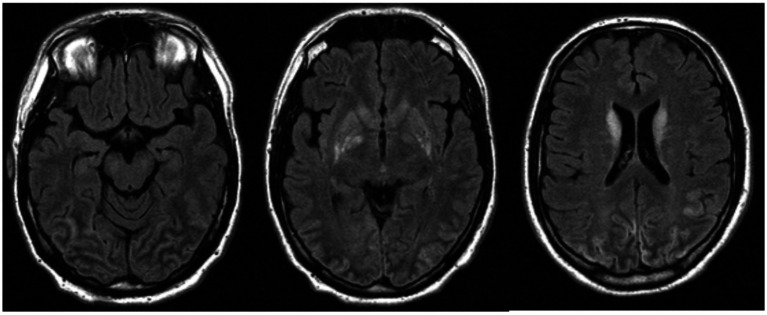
The magnetic resonance image of AC acquired 18 days after the incident, showed edematous alterations and blood–brain barrier dysfunction in the occipital lobes and the posterior-inferior temporal cortex.
